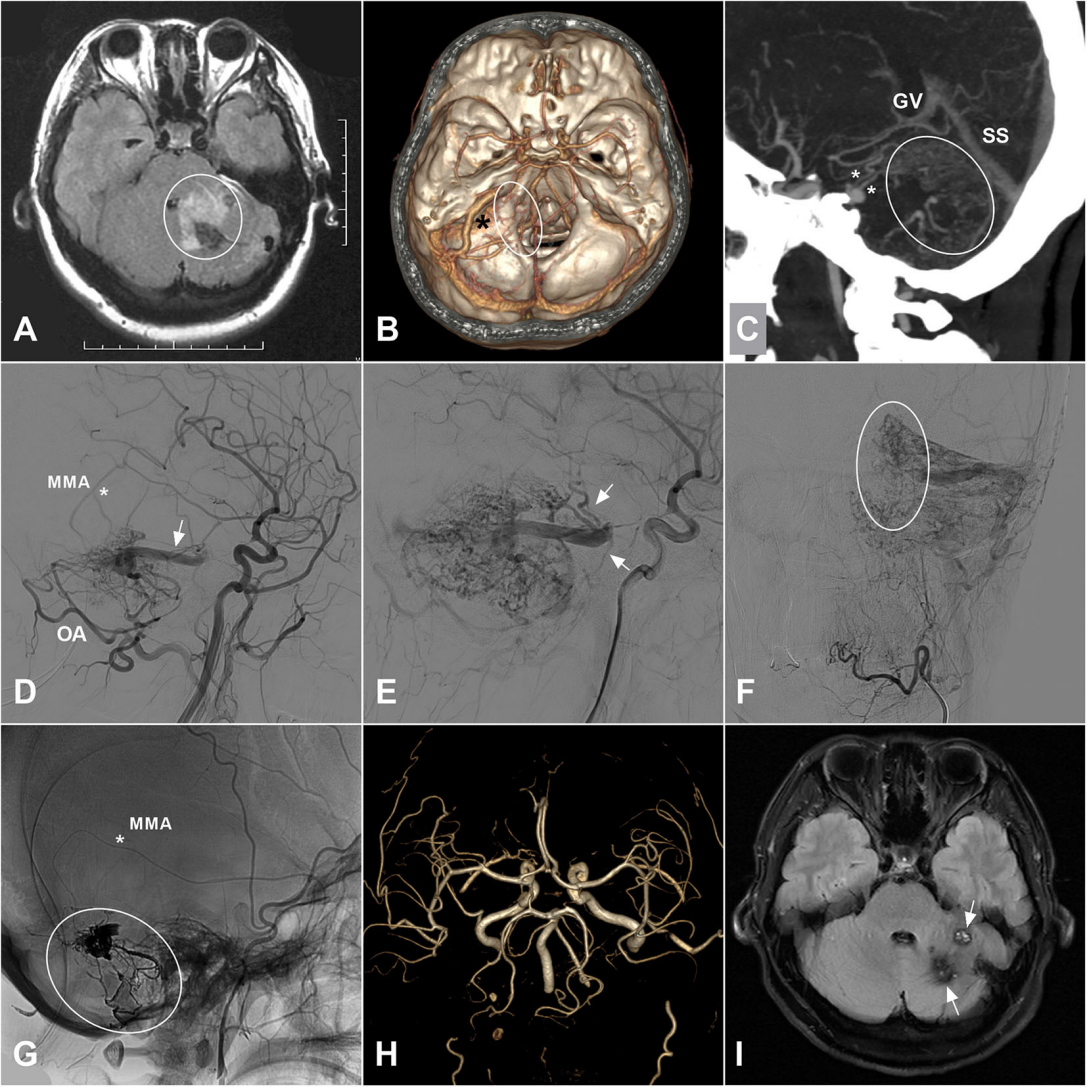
(A) A 35-years-old female was admitted for 3-days history of headache and vomiting. MRI on FLAIR sequence reveals a hyperintense left cerebellar lesion (white circle) with the adjacent brainstem involvement. Besides, vascular flow voids are also noted at the posterior fossa. (B) CTA shows an abnormally enlarged vein (asterisk) draining from the cerebellar surface to the brainstem. And some enlarged veins (ellipse) around the brainstem are also noted. (C) MIP of CTA shows the enlarged draining veins in the cerebellum (ellipse) and around the brainstem (asterisks). (D) Angiogram of the left ECA in lateral view shows a DAVF supplied by the MMA (asterisk) and OA and drained to the deep veins via an enlarged superficial vein (arrow). (E) Angiogram in late arterial phase shows the deep veins (arrow) around the brainstem. (F) Angiogram of the left ECA in anteroposterior view shows enlarged veins in the left cerebellar hemisphere. The ellipse indicates the midline veins. (G) Unsubtracted angiogram shows the DAVF is embolized with Onyx (ellipse) *via* the MMA (asterisk). (H) Follow-up MRA 1 month postoperatively shows disappearance of the DAVF. (I) Follow-up MRI on FLAIR sequence shows remission of the brainstem and cerebellar edema and deposition of hemosiderin (arrow). CTA, computed tomography angiography; DAVF, dural arteriovenous fistula; ECA, external carotid artery; FLAIR, fluid attenuated inversion recovery; MIP, maximum intensity projection; MMA, middle meningeal artery; MRA, magnetic resonance angiography; MRI, magnetic resonance imaging; OA, occipital artery.
